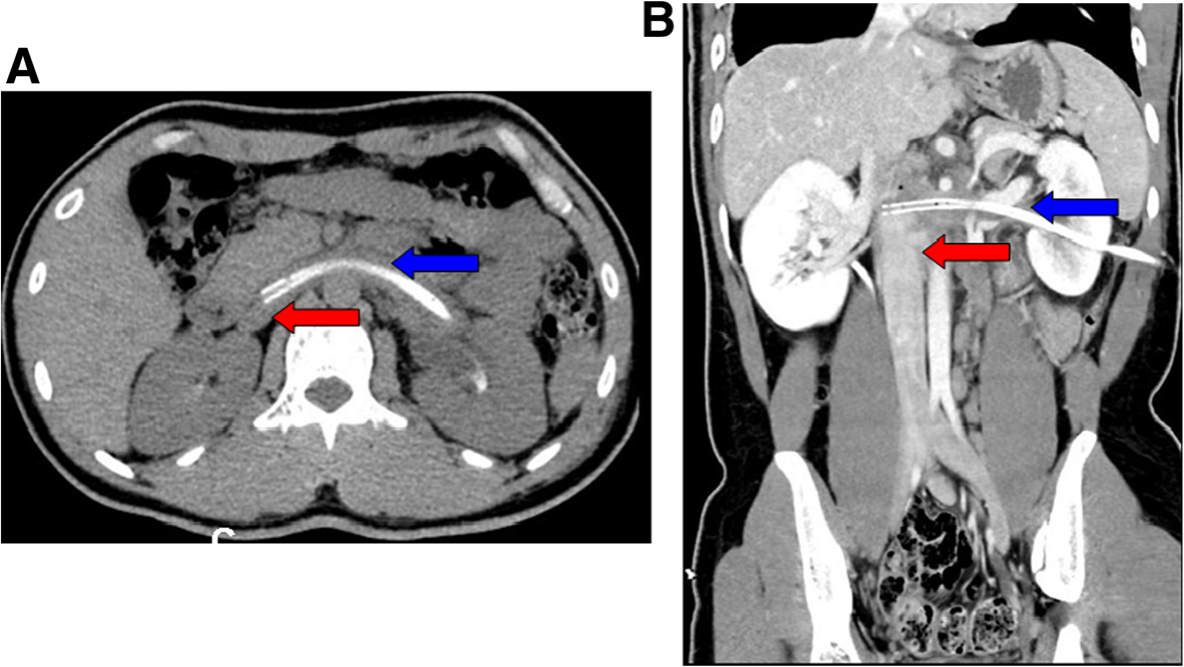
CT revealed the nephrostomy tube piercing into IVC. a Plain of CT scan revealed that nephrostomy catheter (*blue arrow*) had transversed *left* renal vein directly into IVC (*red arrow*). b Enhanced CT scan demonstrated that nephrostomy catheter (*blue arrow*) had transversed *left* renal vein directly into IVC (*red arrow*)
